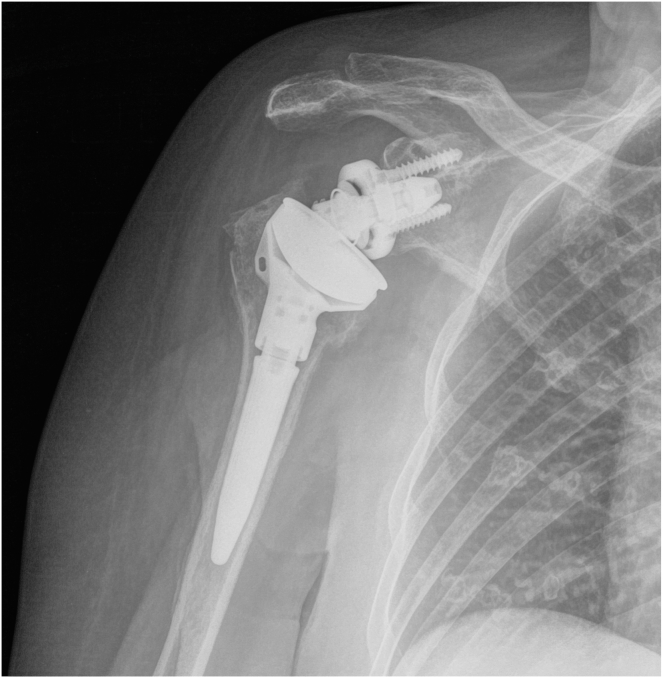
Right* shoulder follow-up radiograph at 31 months postoperatively (83 year old), showing proper healing of both the LT and GT. *LT*, lesser tuberosity; *GT*, greater tuberosity.
